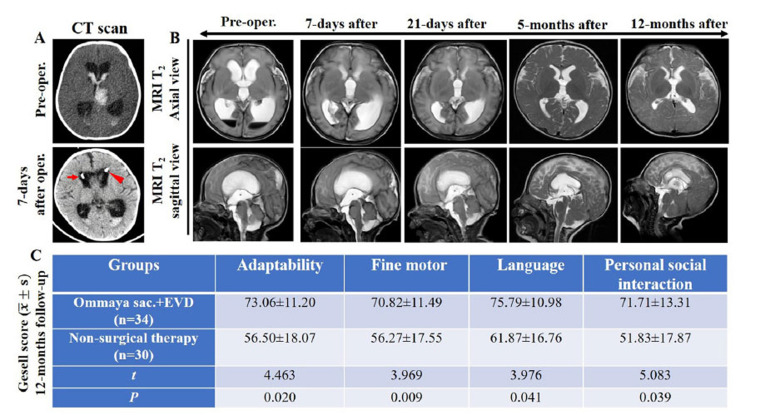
Neurological effects of EVD combined with Ommaya sac in preterm infants with PHH as primary treatment. (A, B): Images of a typical case of PHH in preterm infant before and after surgery (A: CT, B: MRI, red arrow: EVD, red arrowhead: Ommaya sac.). (C): Result of 12 months-Follow-up in terms of adaptability, fine motor, language and personal social interaction (unpublished data). The results were considered significant at a level of *P* < 0.05. It has since been published in the Journal of Army Medical University in Chinese as: Zou Bin, Tang Jun, Zhou Yudong, Chen Xiaobing, Zhao Xueling.: Zou Bin, Tang Jun, Zhou Yudong, Chen Xiaobing, Zhao Xueling, Liang Ping, Yu Zengpeng. Effectiveness of Ommaya sac combined with external ventricular drainage in improving neurological outcome after periventricular-intraventricular hemorrhage in neonates[J]. *J. Army Med. University*, 2022, *44*(17): 1778-1784. DOI: 10.16016/j.2097-0927.202203222).
